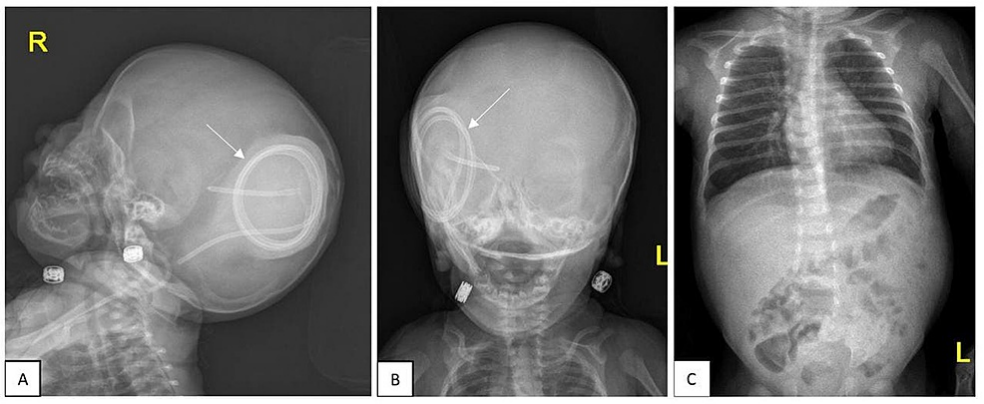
Before the second operation, VP shunt series, lateral (A) and anterior (B) skull view, show upward migration and coiling of the distal catheter. Chest X-ray (C) shows an absence of the distal catheter in the abdomen.
